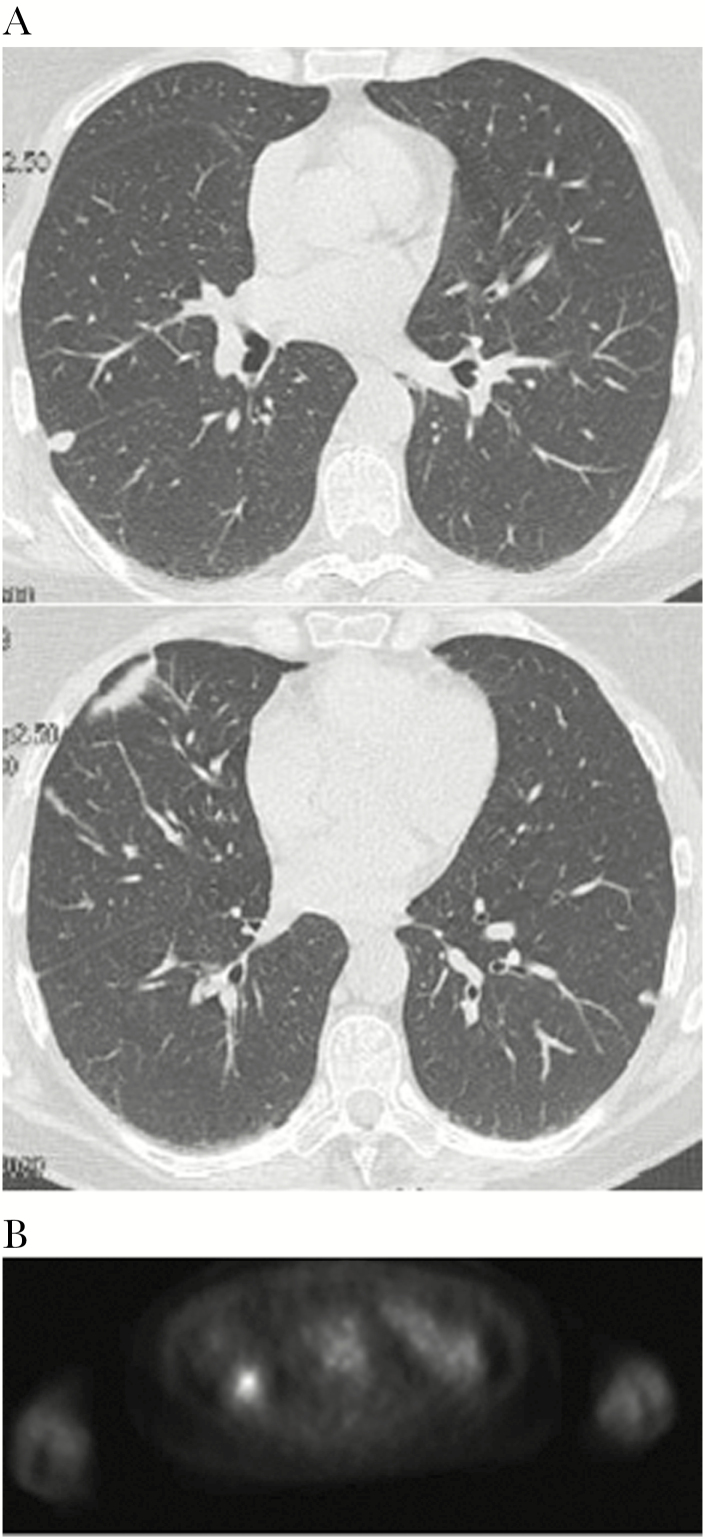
A computed tomography (CT) scan showed multiple nodules in the right lung without lymph node involvement, and the biggest (12 × 5 mm) was in the right lower lobe (RLL) (A). Fluorodeoxyglucose-positron emission tomography/CT confirmed the presence of multiples nodules, showing a low uptake only by the nodule in the RLL (1.7 SUV max) and no uptake in the rest of body (B).
